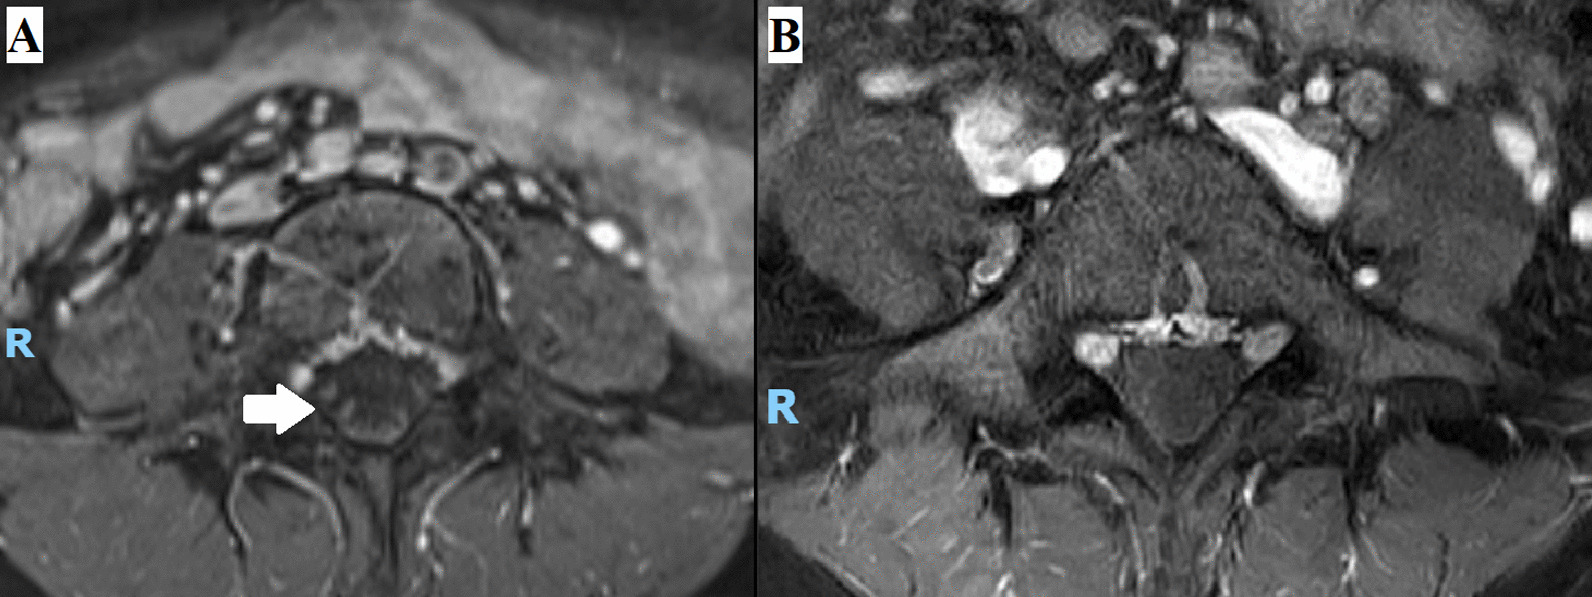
MRI of the lumbar spine. A Axial projection, T1 with gadolinium contrast on the eighth day of symptoms, showing enhancement of the cauda equina nerve roots (arrow) at the L4 vertebral level. B Axial projection, T1 with gadolinium contrast on the 18th week from symptom onset, showing resolution of the nerve root enhancement
